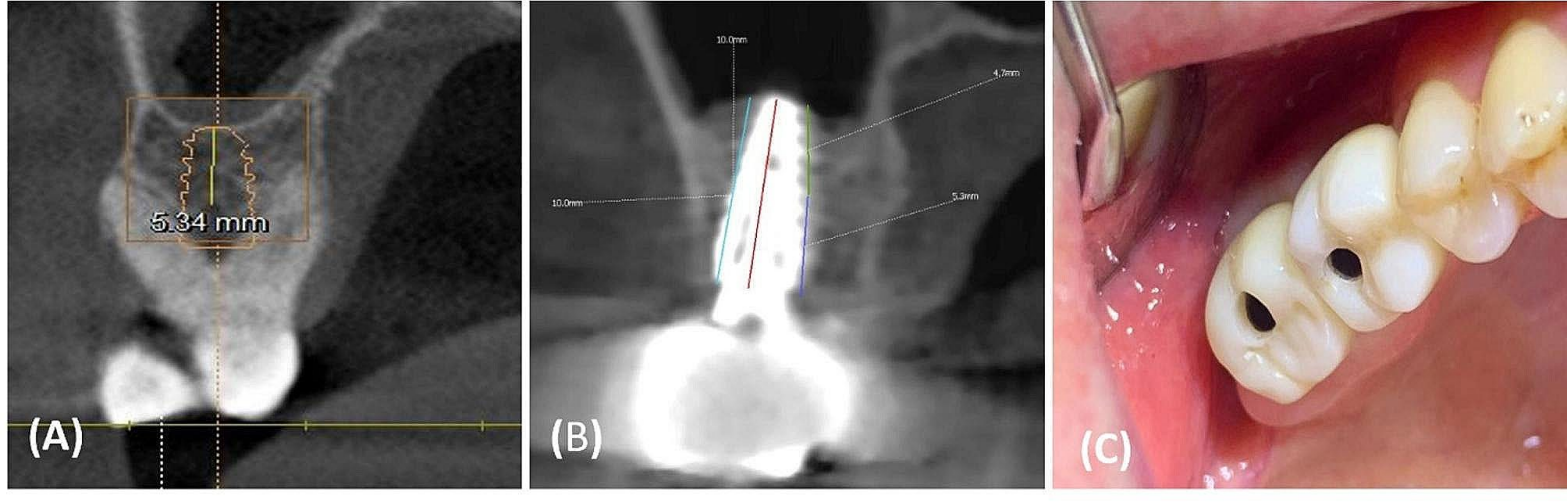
Preoperative and postoperative CBCT scan views of case with freshly extracted socket who needed dental implants in the posterior maxilla and was diagnosed with a reduced vertical bone height the maxillary sinus floor at right upper sex molar; (A) Preoperative coronal view of right upper sex molar, (B) Postoperative coronal view of right upper sex molar at one year after maxillary sinus lifting and implant insertion, (C) Postoperative clinical view
